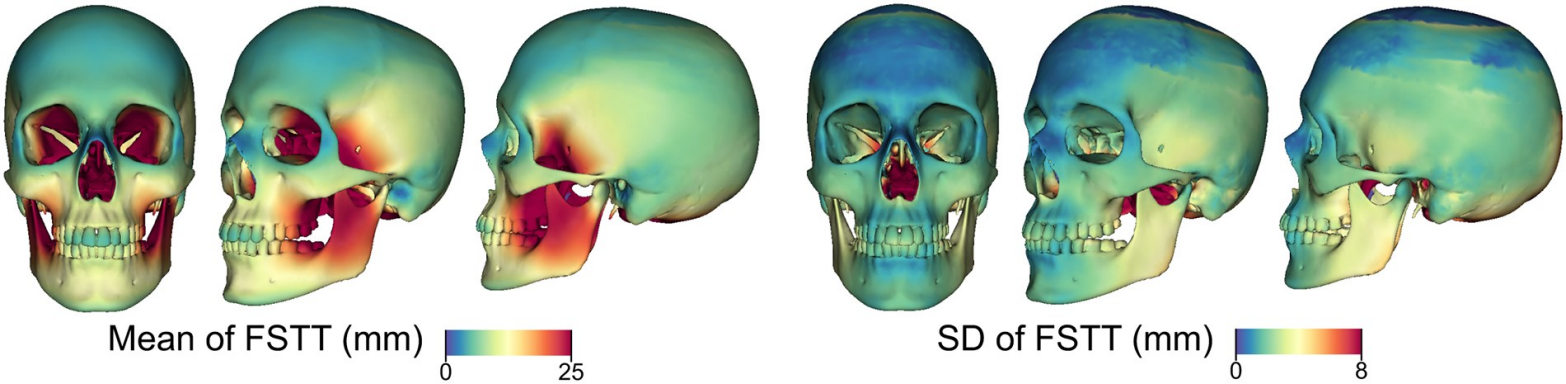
Statistic of the FSTT on a mean skull. Mean and standard deviation of FSTT computed from the 43 CT scans.
